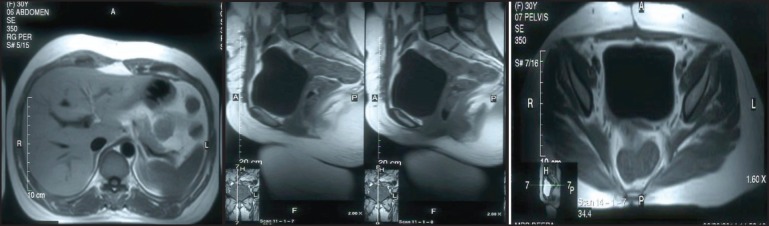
MRI of abdomen and pelvis (sagittal and transverse view) not showing any abnormalities after the completion of six cycles of adjuvant chemotherapy.
